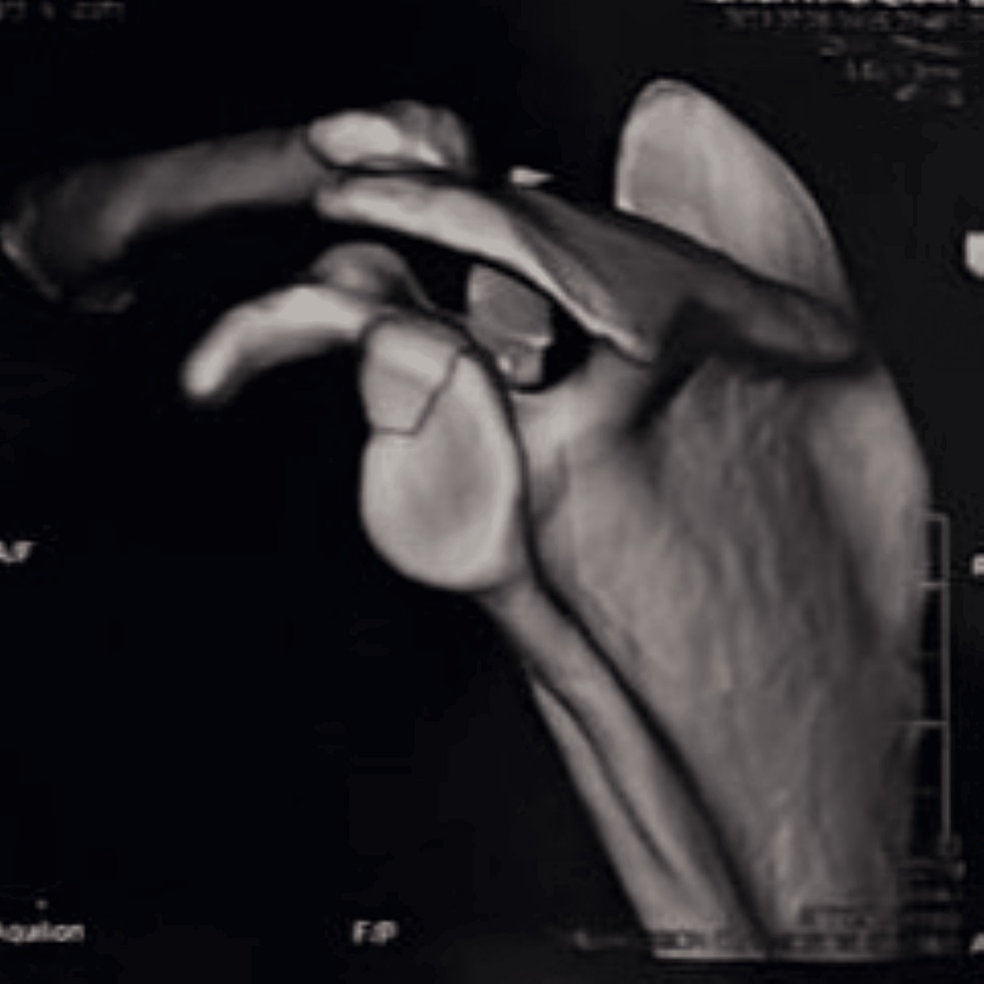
Post 20-week follow-up 3D CT scan
